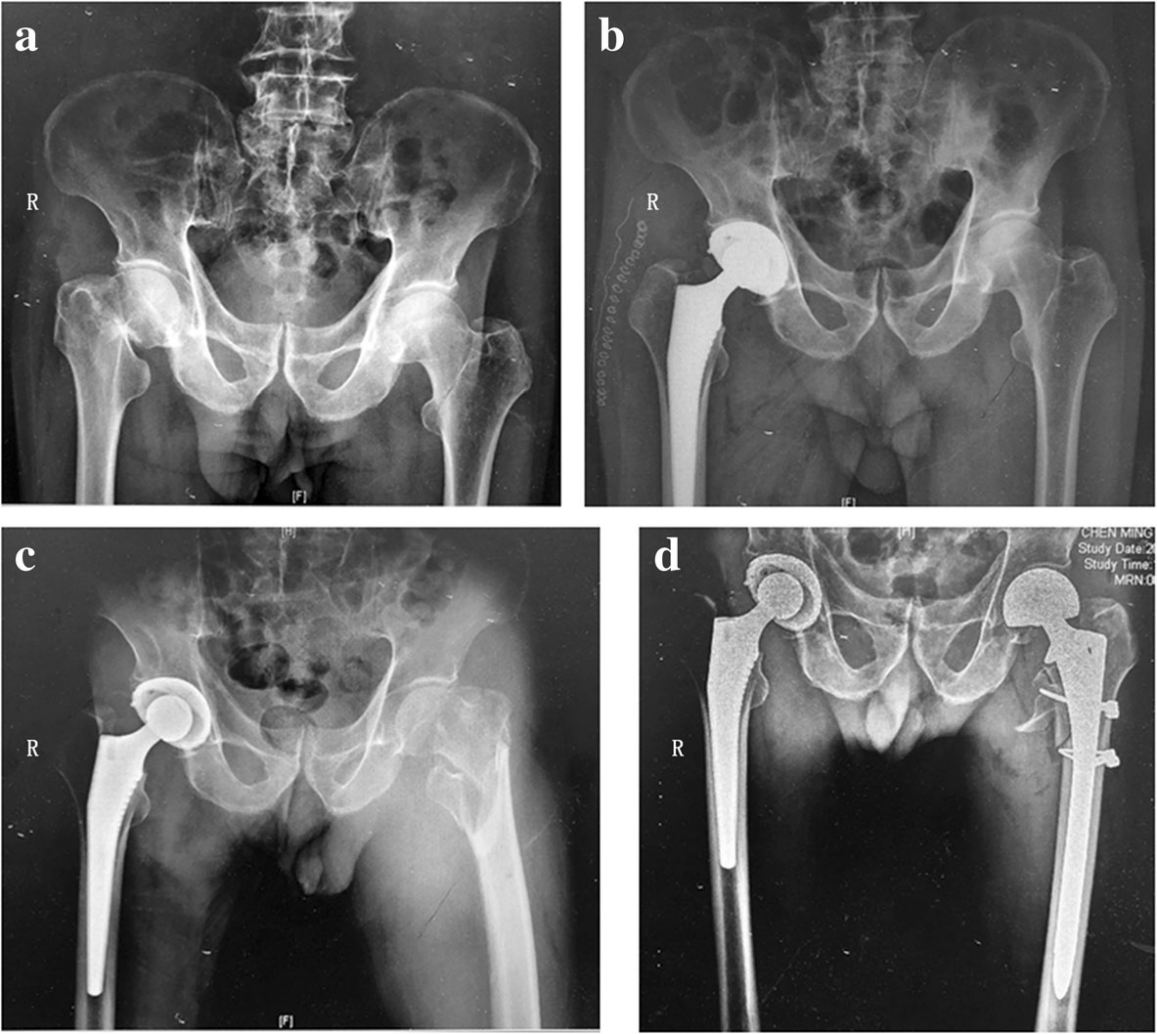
An 81-year-old male patient with sequential hip fractures. a Antero-posterior pelvic X-ray showing femoral neck fracture in the right hip upon admission to the hospital. b The patient was treated in an optimized practice for superaged patients with hip fracture, including total hip replacement within 48 hours after injury. c A fall 1 month later resulted in a femoral intertrochanteric fracture of the left hip. d The patient was managed with damage control principles and enhanced recovery after surgery, including femoral head replacement within 48 hours after injury
